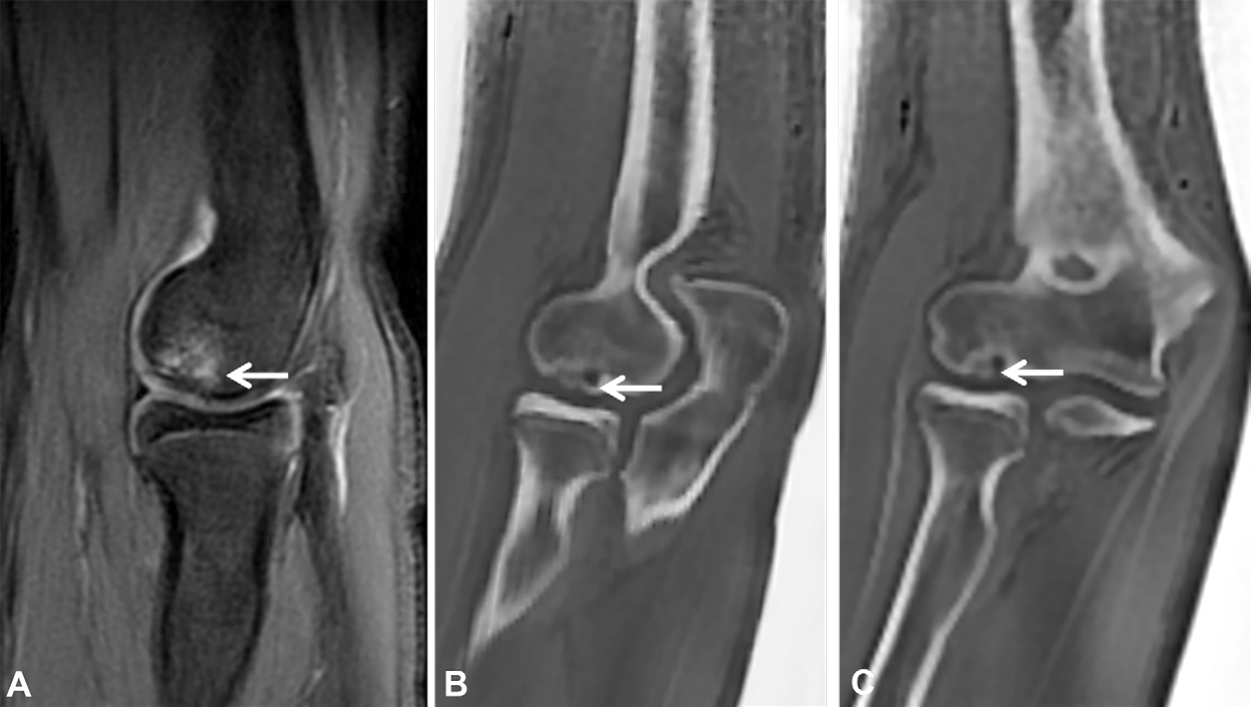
A 15-year-old female gymnast with pain in the elbow. (A) Sagittal T2 fat-suppressed MRI demonstrates subchondral bone marrow edema (arrow) in the capitellum accompanied with thinning of the overlying articular cartilage. (B, C) Sagittal and coronal ZTE images provide enhanced visualization of the articular surface, depicting contour irregularity and a subtle bony fragment (arrows).
